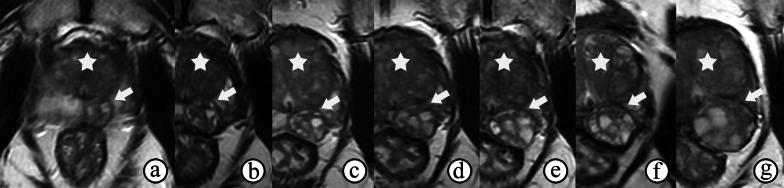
BPH in a 66-year-old man with an elevated serum PSA level fluctuating from 4 to 10 ng/mL. a–g Axial T2-weighted images captured in Jan 2010 (size of 1.3 cm), May 2012, Mar 2013, Dec 2013, Feb 2015, May 2015, and Jul 2019 (size of 2.6 cm) show a gradually increasing nodule with heterogeneous hyperintensity and a well-defined capsule in the left peripheral zone (arrow), the size of which increases with the progress of BPH in the TZ (☆)
